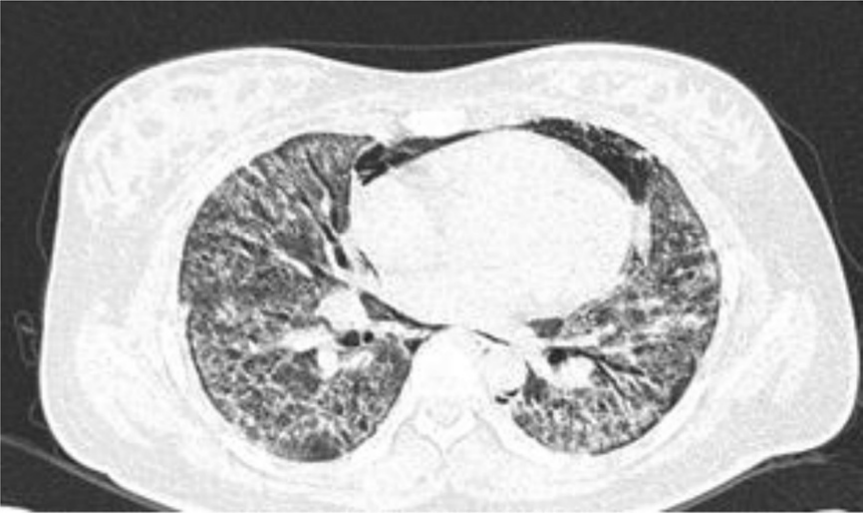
Chest computed tomography (CT) image showing pneumomediastinum and bilateral diffuse ground glass opacity (GGO) lung infiltration in the peribronchial area in both lower lung fields in the initial phase observed in a fatal case (28 years old and pregnant female).
